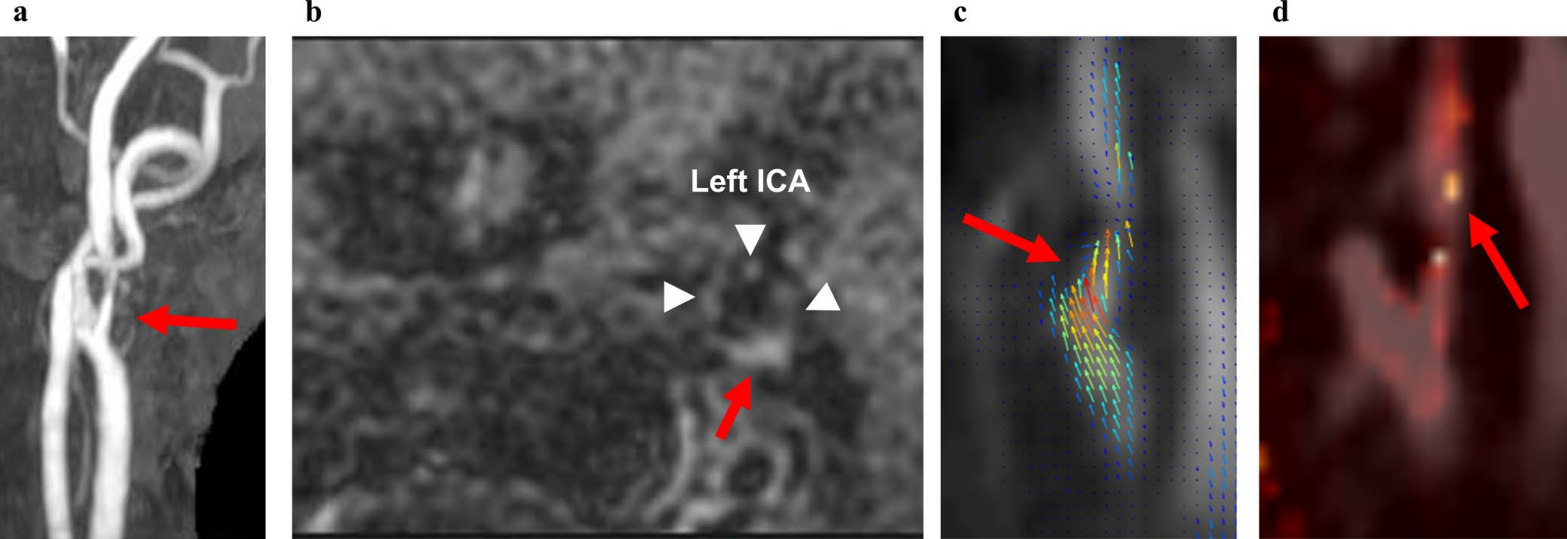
A representative case (78‐year‐old female with 72% left ICA stenosis). (a) TOF‐MRA MIP image; (b) T1BB image; (c) 4D flow MRI (vector overlay map); and (d) 4D flow MRI (TKE map). A 72% stenosis is observed at the origin of the left ICA (a, arrow). A high‐intensity plaque is identified at the corresponding site on T1BB (b, arrow; arrowheads indicate the left ICA). Jet flow is observed proximal and distal to the stenosis (c, arrow). TKE is markedly elevated distal to the stenosis, suggesting the presence of post‐stenotic turbulence (d, arrow). Although detailed assessment of local flow at the stenotic site is limited due to spatial resolution, the observed turbulence distal to the stenosis likely reflects disturbed flow at the stenotic segment. This finding may indicate a potential association between post‐stenotic turbulence and the development of unstable plaque. ICA = internal carotid artery, TKE = turbulent kinetic energy.
